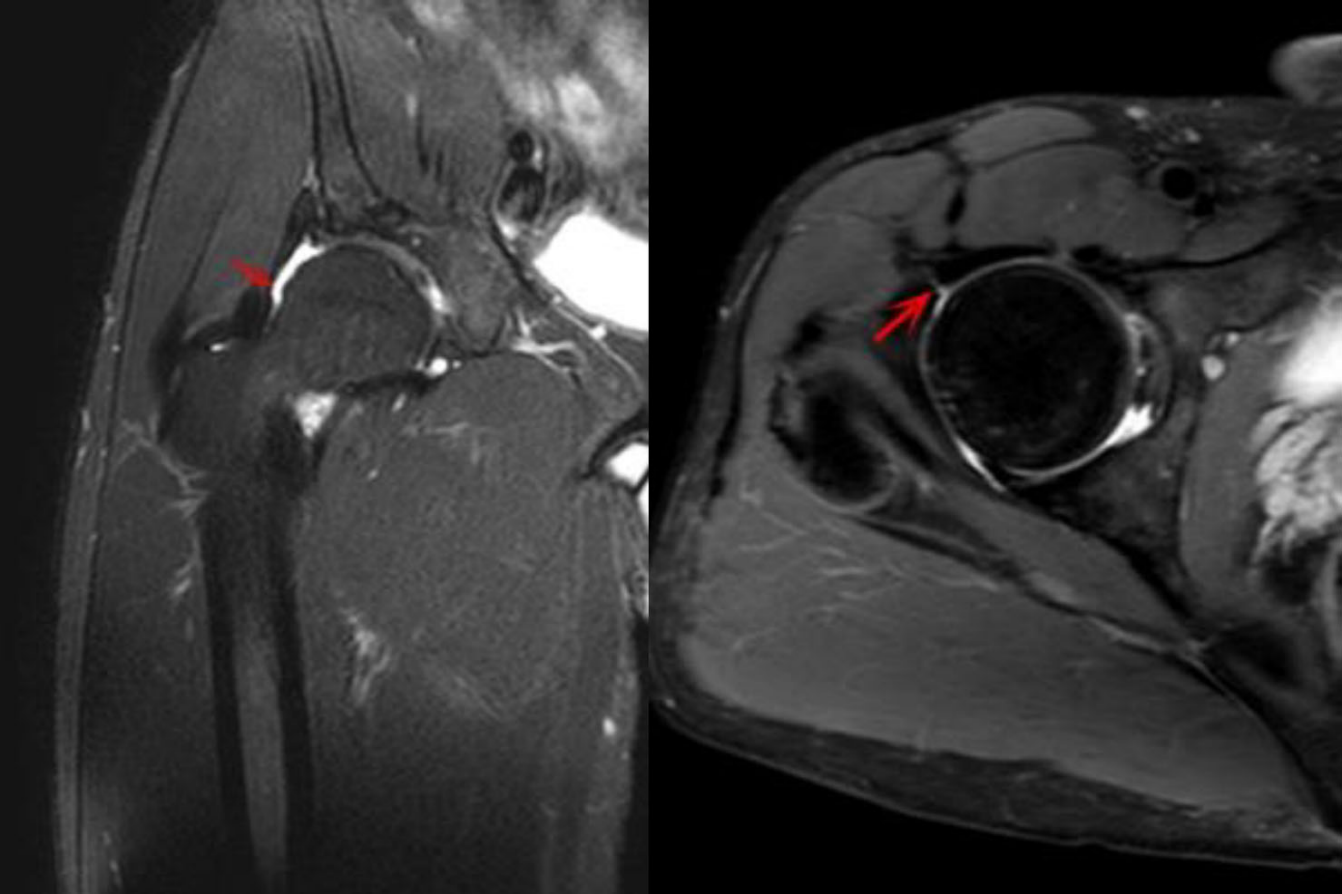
MRI shows 24 months after hip arthroscopy, the capsule has not healed (red arrow).
